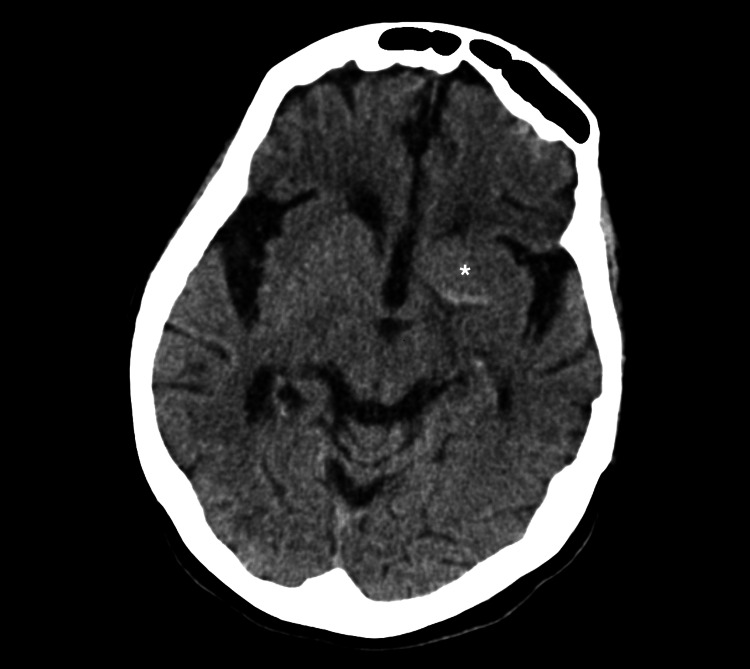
Cranial CT angiography. Absence of contrast column filling with hyperdensity of the left proximal middle cerebral artery suggesting endoluminal thrombus in the M1 segment (*).
